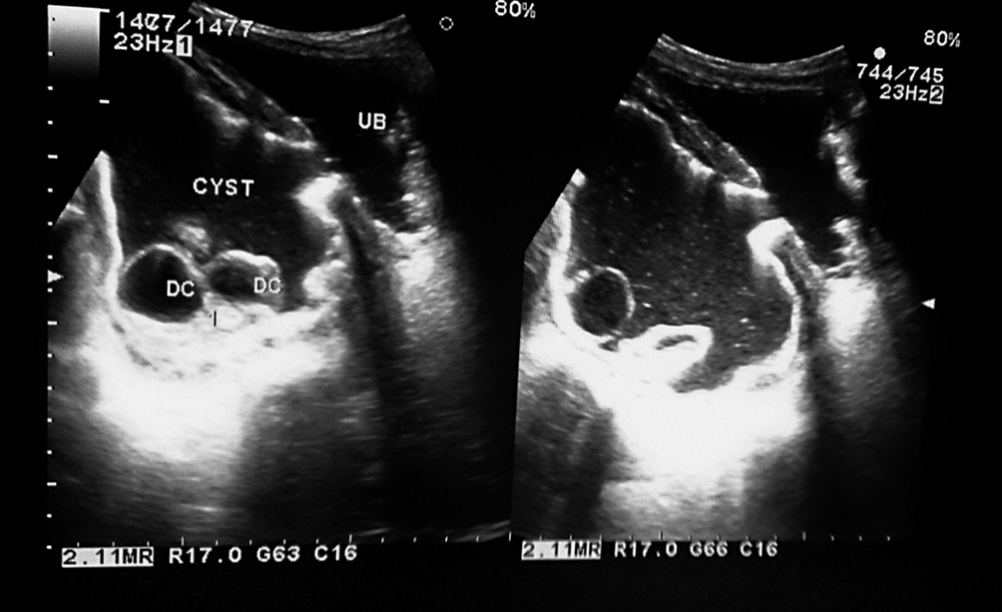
Longitudinal ultrasonographic scan showing fistulous communication between the urinary bladder (UB) and the hydatid cyst containing free daughter cysts (DC).
